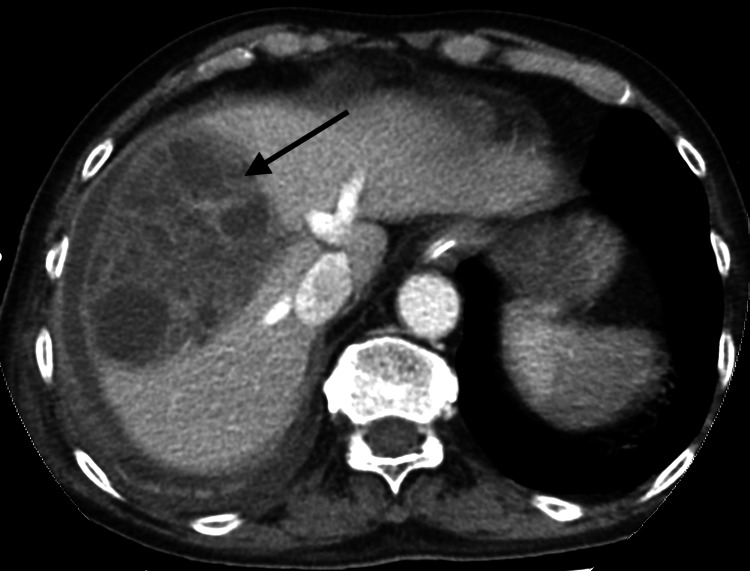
Axial view of computed tomography of the abdomen showing right liver lobe abscess (black arrow) with subcapsular liver collection
